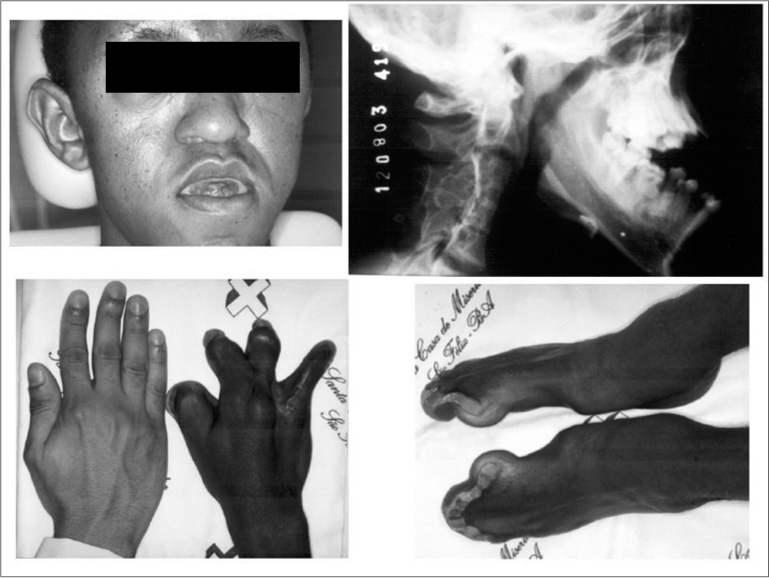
Medical and radiographic findings.
